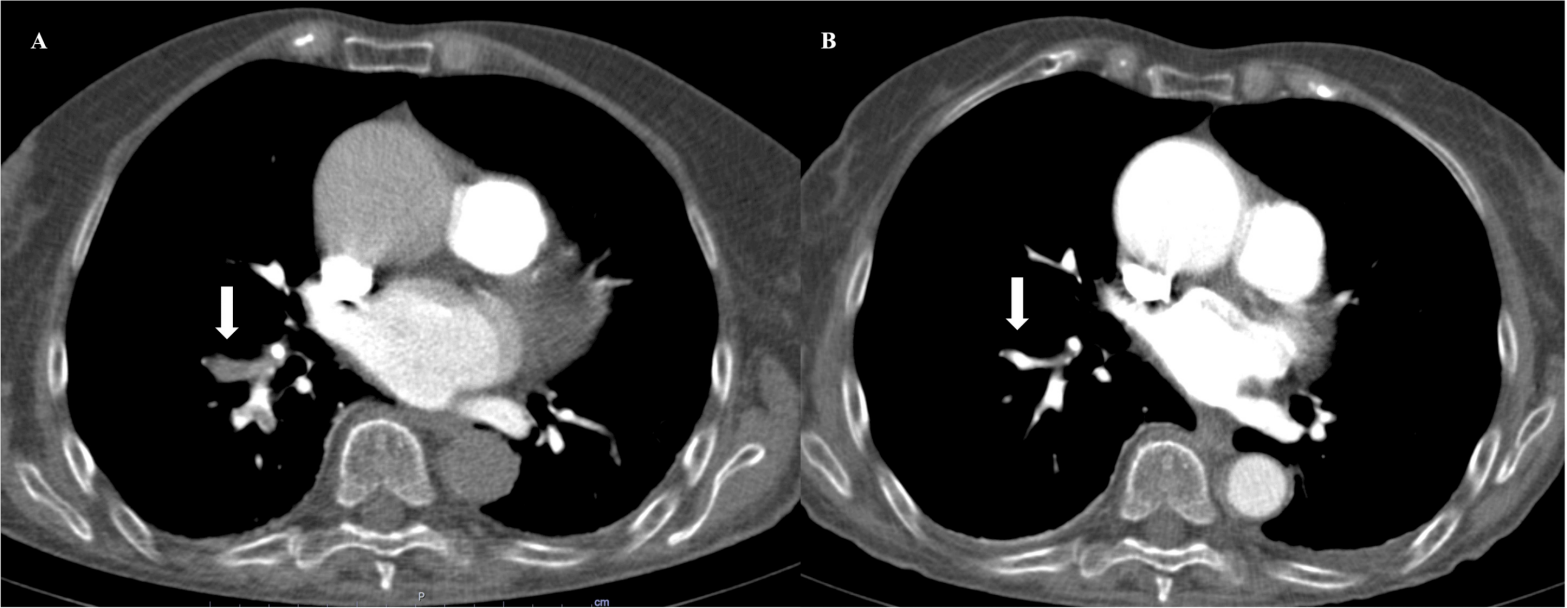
CT angiography of the chest on the third day of hospitalization. CT angiography of the chest on the third day of hospitalization (A) showing PE in the anterior, lateral, and posterior segmental branches of the right lower lobe (arrow). (B) Seven days after the start of hypocoagulation, permeabilization was observed in the segments where the absence of opacification had previously been identified (arrow).
